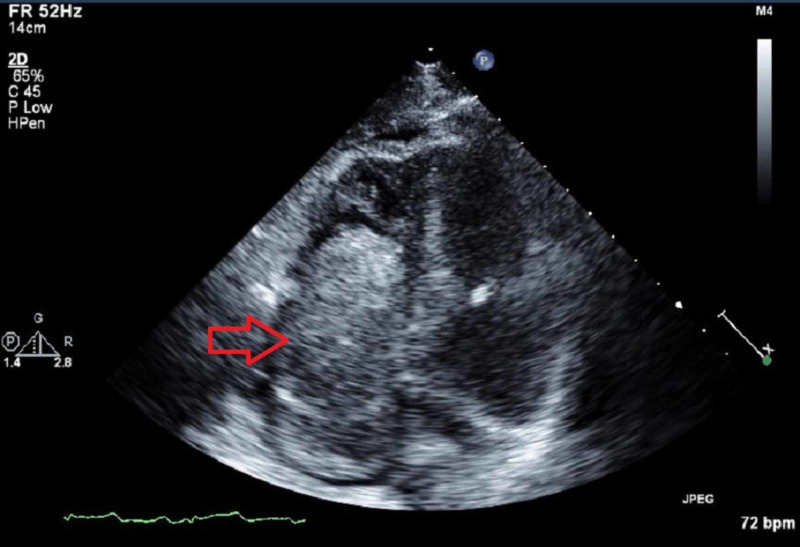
Apical four-chamber transthoracic echocardiogram view showing the mass in the right side of the heart.
